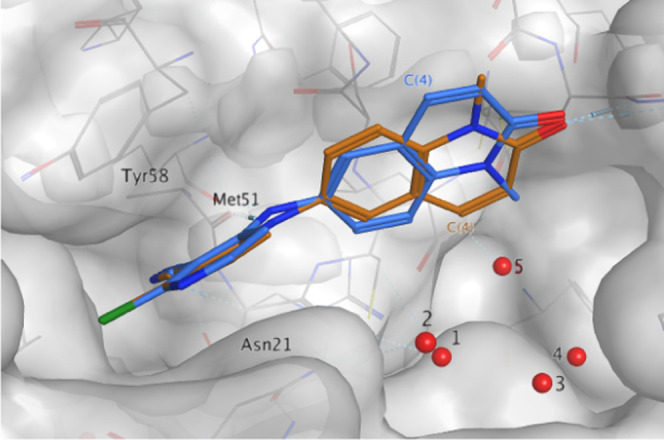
X-ray structure of 2 (PDB: 7OKE, 1.48 Å resolution)
